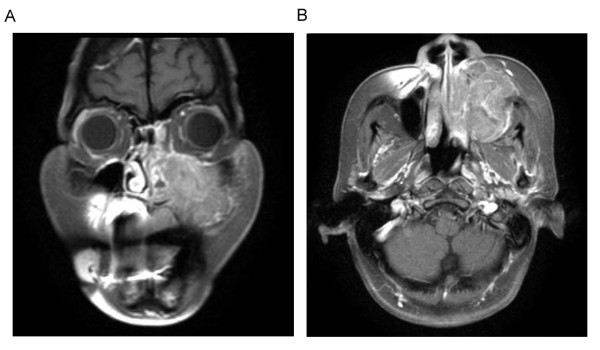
Post-gadolinium contrast-enhanced T1-weighted coronal magnetic resonance imaging (MRI), with fat saturation, showed an enhancing and destructive mass with indistinct borders in left maxillary sinus. (A) Coronal view. (B) Axial view.
